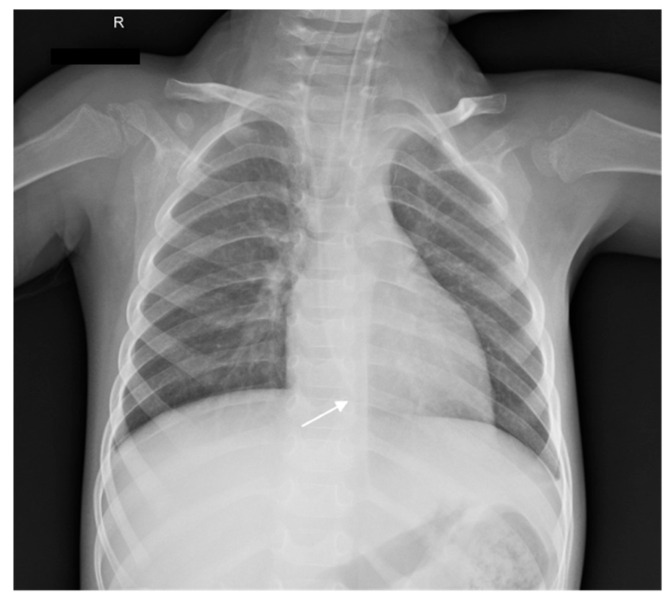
Chest radiography of Patient 2 upon admission showing the intracardiac position of the catheter tip (white arrow). R: right.
